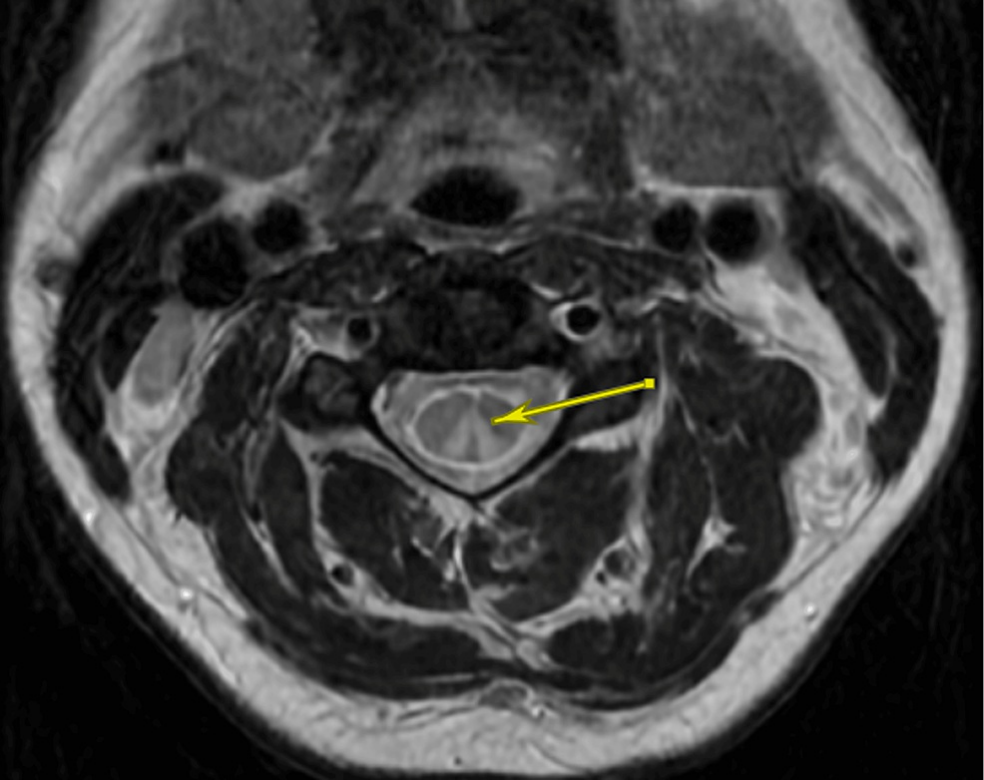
T2 hyperintensity most prominent in the dorsal columns suggestive of subacute combined degeneration. MRI of the cervical spine (Axial, T2 fast spin echo) at the C2-C3 level showing T2 hyperintensity within the dorsal columns.
